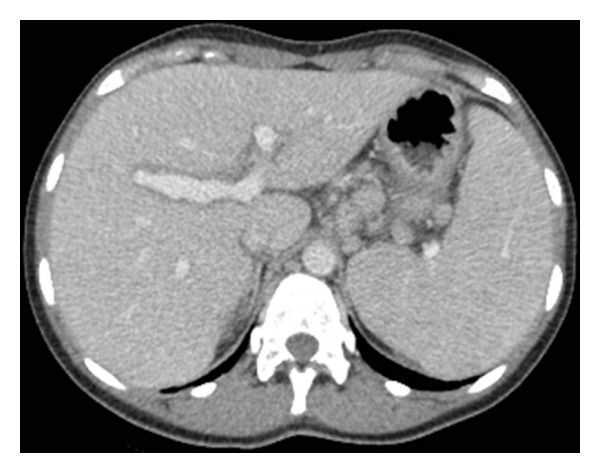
Abdominal CT showing mild liver and spleen enlargement.
